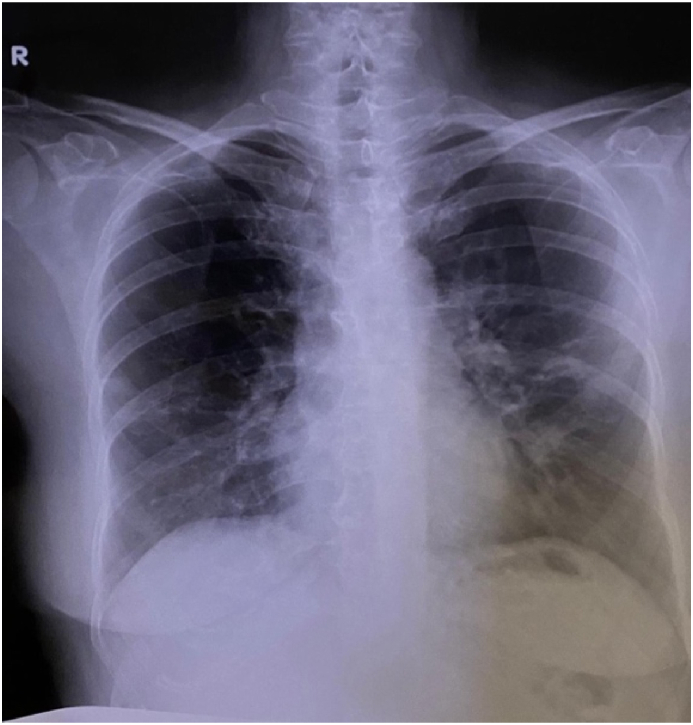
Chest X-ray (may 14th,2022).
